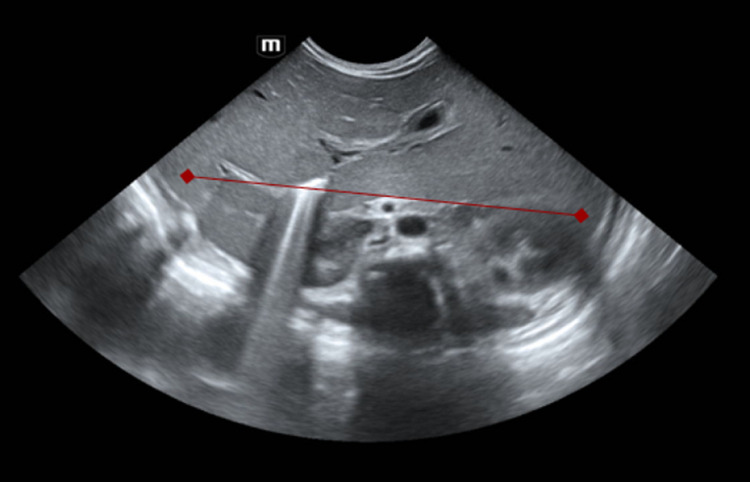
abdomen ultrasound shows the liver is enlarged occupying both hypochondria; no focal lesion and hepatic veins and inferior vena cava are not congested; portal vein is not seen dilated
